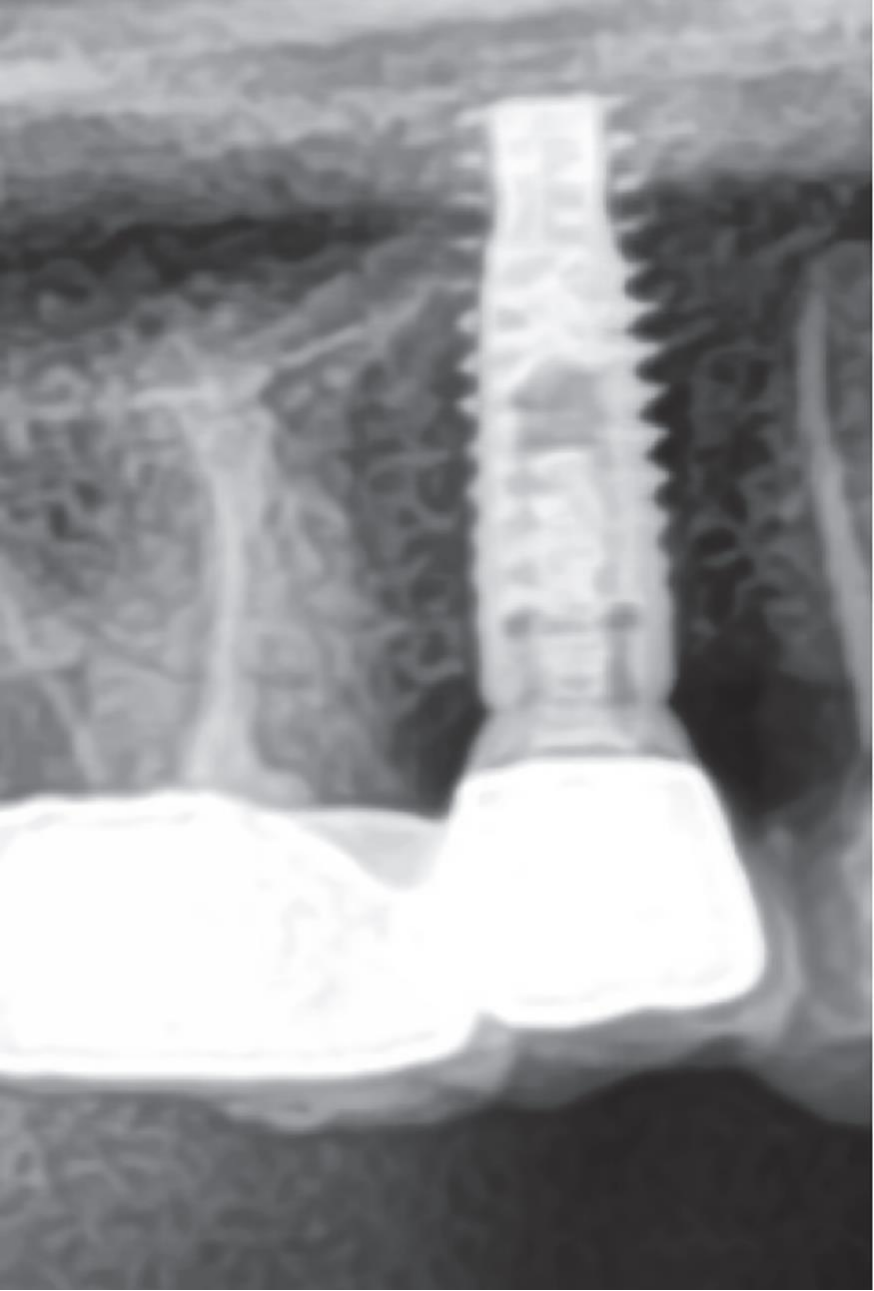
Preoperative radiographic image showing the implant with adequate peri‐implant bone support and no evidence of marginal bone loss prior to crown removal.
